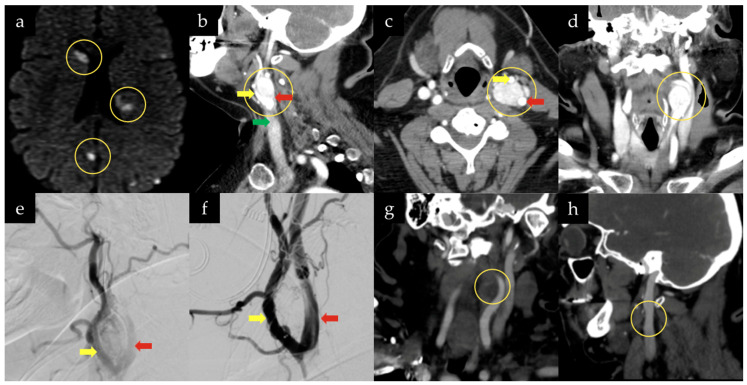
Multimodal imaging of a 51-year-old woman with a left carotid body tumor treated with preoperative embolization and surgical resection. (a) Diffusion-weighted MRI demonstrates multifocal areas of restricted diffusion (yellow circles) within the supratentorial cerebral hemispheres, more prominent on the left, including involvement of the caudate head and putamen, consistent with acute multifocal ischemic infarctions without evidence of intracranial hemorrhage. Sagittal (b), axial (c), and coronal (d) contrast-enhanced CT angiography of the neck demonstrate a hypervascular mass centered at the carotid bifurcation (yellow circle) with a circumferential relationship to the cervical carotid vasculature and delineate the craniocaudal extent of the lesion. (e) Preoperative digital subtraction angiography demonstrates marked tumor vascularity supplied predominantly by branches of the external carotid artery, with substantial reduction in tumor blush following selective embolization (f) and preserved patency of the internal and external carotid arteries. Postoperative coronal (g) and sagittal (h) CT angiography confirm complete tumor resection without residual or recurrent lesion and preservation of carotid artery anatomy (yellow circles). (ICA, red arrow; ECA, yellow arrow; CCA, green arrow).
